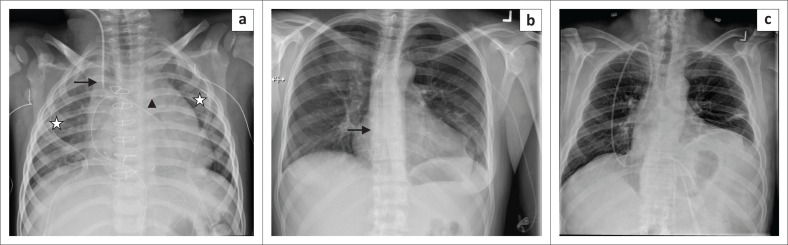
(a) Chest radiograph showing a right central venous catheter (arrow) with its end located in the SVC. In addition, bilateral intercostal drainage tubes (stars) and mediastinal drainage tube (arrow head) are noted; (b) chest radiograph showing a left-sided, peripherally inserted central catheter with its tip (arrow) in the right atrium; (c) a tunnelled right central venous catheter.
